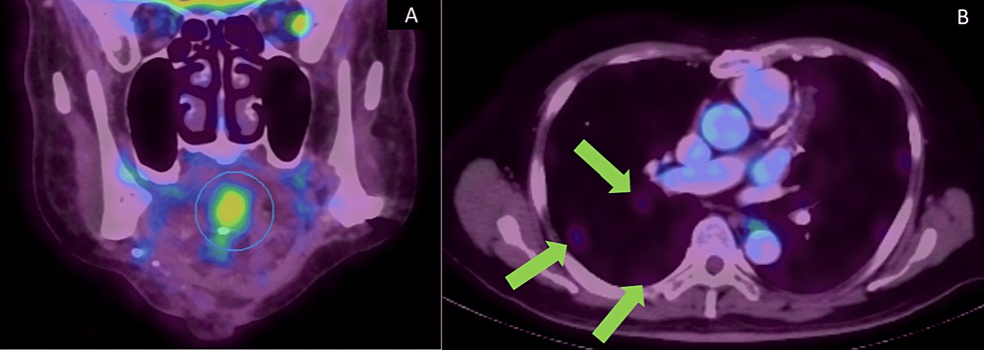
Photograph shows PET-CT image (A) of the recurrent tumor on the base of the tongue (blue circle) and (B) three metastatic nodules in the right lung (green arrows) PET-CT: positron emission tomography-computed tomography
